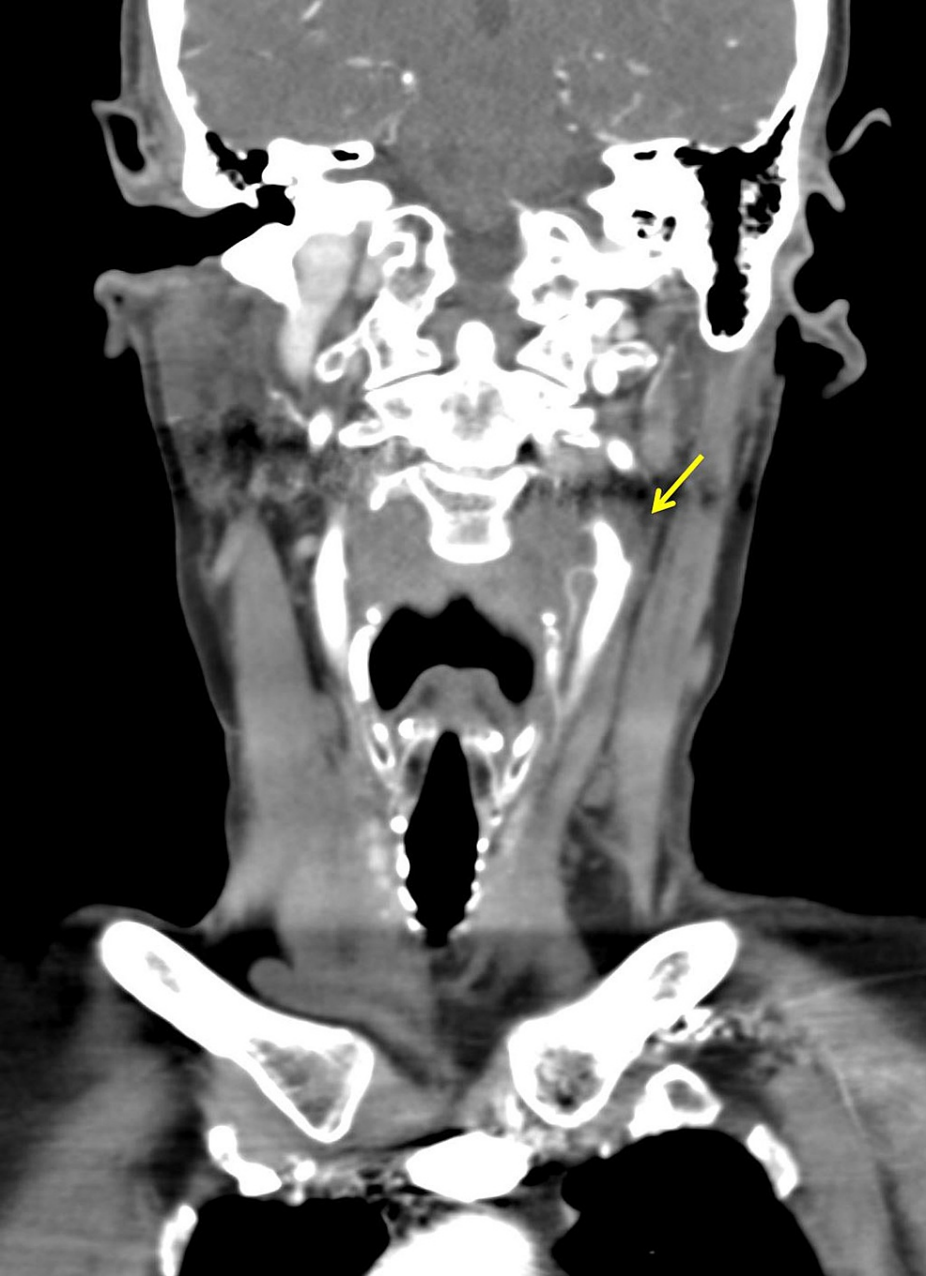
Coronal contrast-enhanced CT image demonstrating inflammatory soft tissue (arrow) surrounding the left distal common carotid trunk and carotid bifurcation without notable luminal narrowing.
